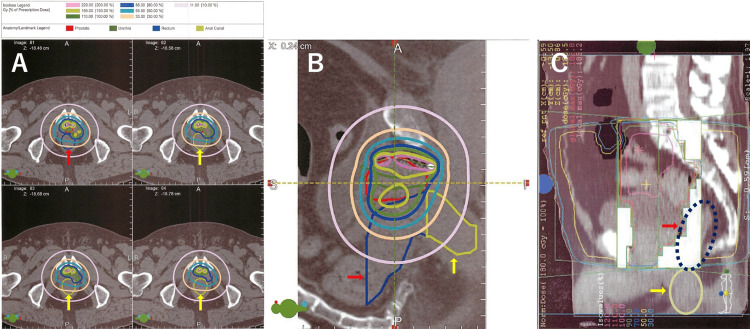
Post-implant and external beam radiotherapy plans of Case 2 (A) Axial CT slices showing the contoured rectum (red arrows) and anal canal (yellow arrows). (B) Sagittal reconstruction with isodose distribution. (C) Archived DVH printout of the EBRT component (45 Gy). Although digital DVH data were not preserved due to the older treatment system, this figure demonstrates the delivered external beam plan in relation to the anal canal region. DVH: dose-volume histogram; EBRT: external beam radiotherapy
